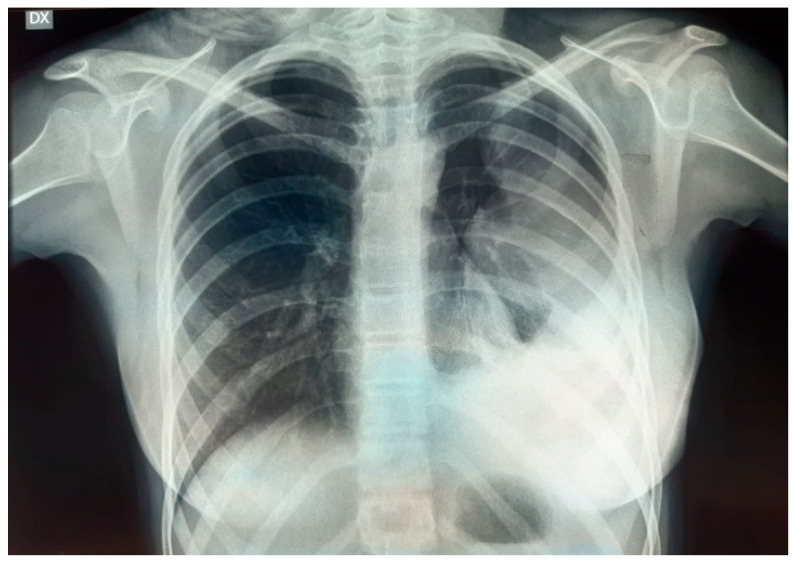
Chest X-ray at presentation.
